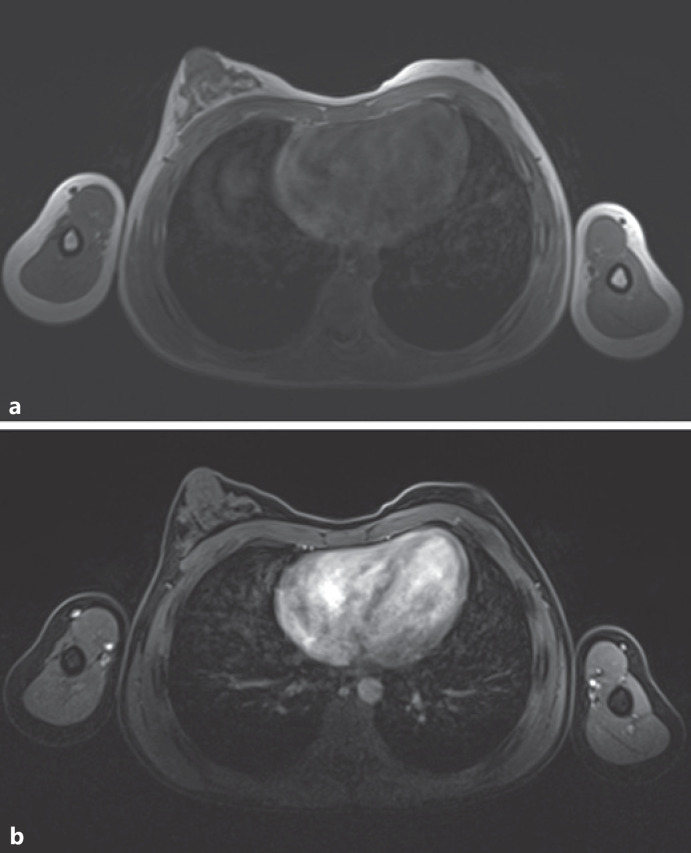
Magnetic resonance imaging (MRI) of the breast in prone position. a Transversal T1-weighted image: depicting clear lateral asymmetry of the glandular tissue in favor of the right side. b Slice (exemplary) of T1-weighted sequence with fat saturation after i.v. contrast agent administration: no revelation of any suspicious enhancement.
